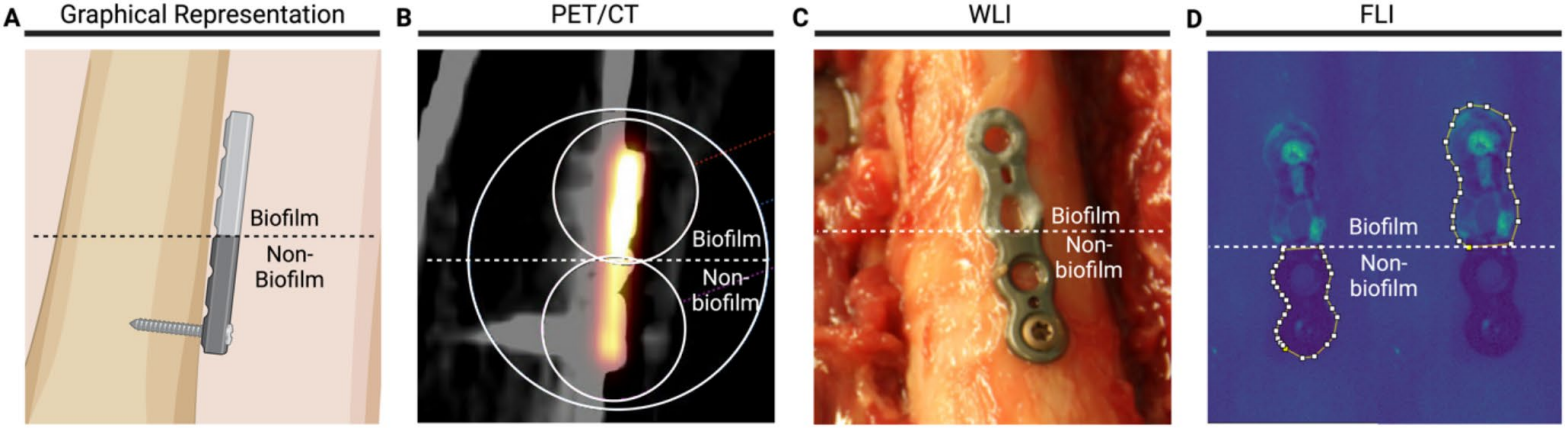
PET imaging and FLI of an osteosynthesis plate mounted on a human femur. (A): Graphical representation of femur and osteosynthesis plate. The non-biofilm side of the implant is oriented towards the bottom of the image, and the biofilm-covered side is oriented towards the top of the image. (B): PET/CT image of the osteosynthesis plate. The screw can be seen in the lower half of the image, protruding from right to left into the femoral cortex. VOIs are shown for the biofilm-covered side, non-biofilm side, and entire osteosynthesis plate. The biofilm-covered side (top) visually shows the highest tracer activity. (C): White light image (WLI) of the osteosynthesis plate in situ with biofilm present on the top half of the implant. (D): FLI of the osteosynthesis plate. The yellow line indicates the regions of ROIs for the non-biofilm side and biofilm side, respectively
